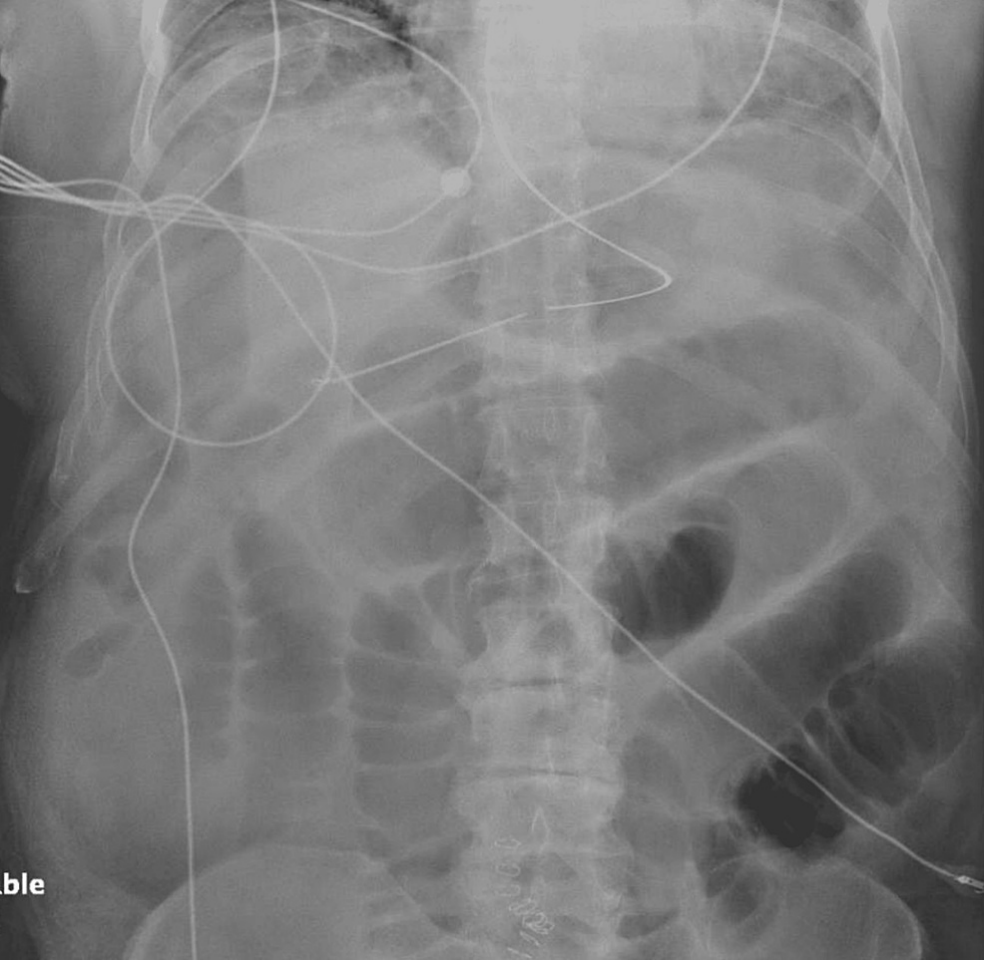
X-ray of the abdomen showing dilated small bowel loops in the mid and upper abdomen with a relative paucity of bowel gas in the colon.
